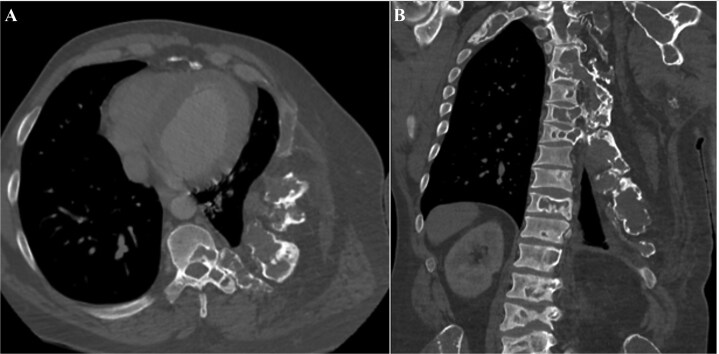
CT of the chest. (A) Transverse view of CT of the chest. (B) Coronal view of CT of the chest showing left-hemi thorax grossly reduced in volume with the expansile fibrous lesions involving multiple ribs, the left shoulder girdle, and the left half of the dorsal vertebra.
